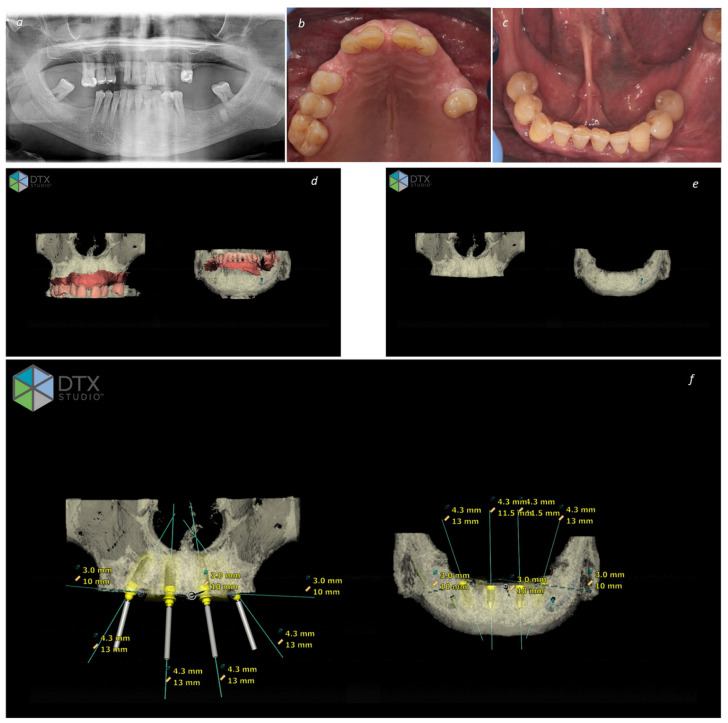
Planning a full-arch bimaxillary rehabilitation through the All-on-4 Concept: (a) Pre-treatment orthopantomography; (b) Pre-treatment intraoral photograph of the maxilla; (c) pre-treatment intraoral photograph of the mandible; (d) DTX Studio image exhibiting the pre-treatment condition in the maxilla and mandible with superimposition of CBCT and Intraoral Scanner (smart fusion); (e) DTX Studio image of the planning exhibiting the bone regularization to achieve a stable platform for implant insertion in the maxilla and mandible; (f) DTX Studio image of the final planning exhibiting the implant planning (diameter and length) in the maxilla and mandible.
